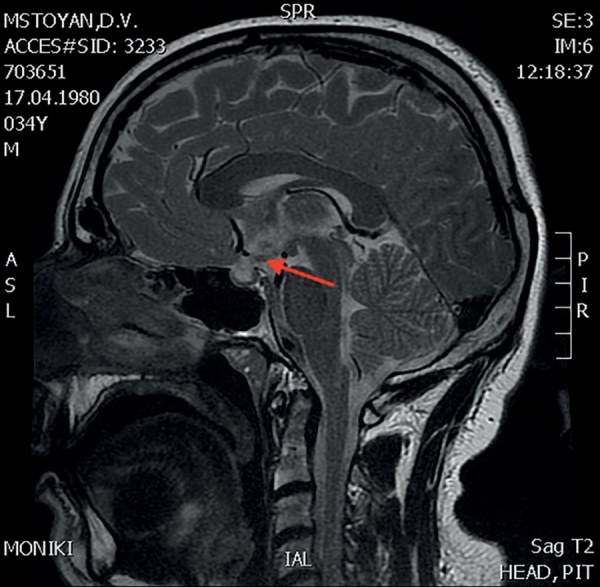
Рисунок 1. МРТ головного мозга с к/у от 03.10.2014г.(3,0 Тл)Саркоидная гранулема в проекции гипоталамуса, сосцевидных тел и прилежащих отделов воронки (указано стрелкой). Figure 1. MRI of the brain with c/c dated 03.10.2014 (3.0 T)Sarcoid granuloma in the projection of the hypothalamus, mastoid bodies and adjacent parts of the infundibulum (indicated by an arrow).
